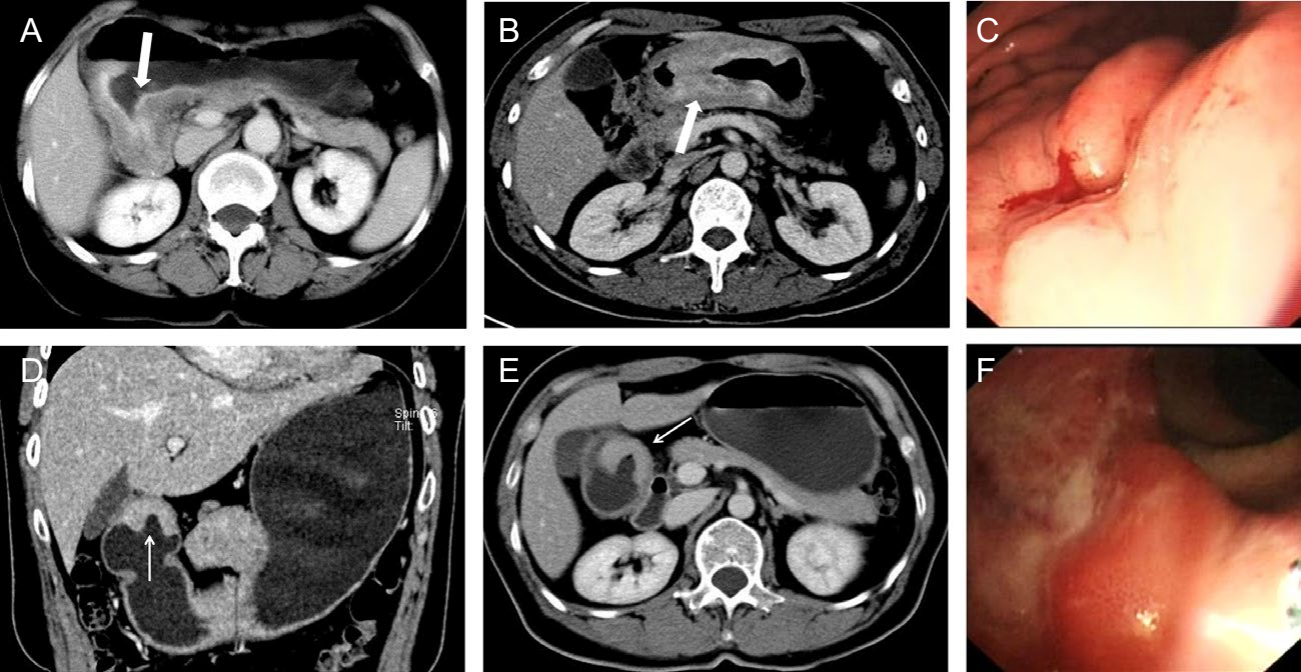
Contrast‐enhanced computed tomography images of advanced gastric carcinoma. (A, B) Two contrast‐enhanced CT images in different patients with advanced SRC. Contrast‐enhanced CT scan shows diffuse gastric wall thickening with strongly enhancement. The layered and heterogeneous‐enhancement pattern is shown. (C) Endoscopic image (same patient in B) of the lesion shows a diffusely infiltrating lesion. (D–F) 55‐year‐old man with NSRC. Contrast‐enhanced CT scan and coronal reconstruction show focal gastric wall thickening mainly of the enhancing thickened inner layer (arrow). The homogeneous‐enhancement pattern is shown. Endoscopic image of the lesion shows an ulcer lesion located in the gastric antrum.
